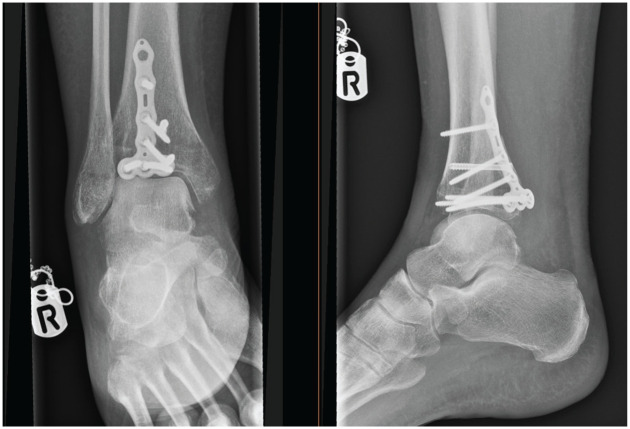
Radiograph showing (Volition) type III (posterior tibial plate) in situ.
